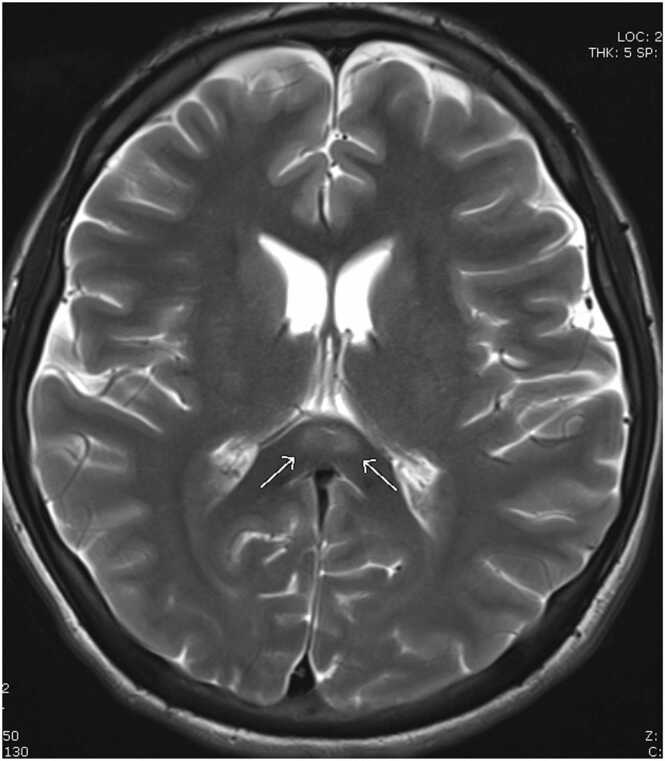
Oval shaped corpus callosum lesion with diffusion restriction, high T2 and FLAIR signal without enhancement or hemorrhagic component on SWI consistent with cytotoxic lesion of corpus callosum (Transient splenial lesion).
